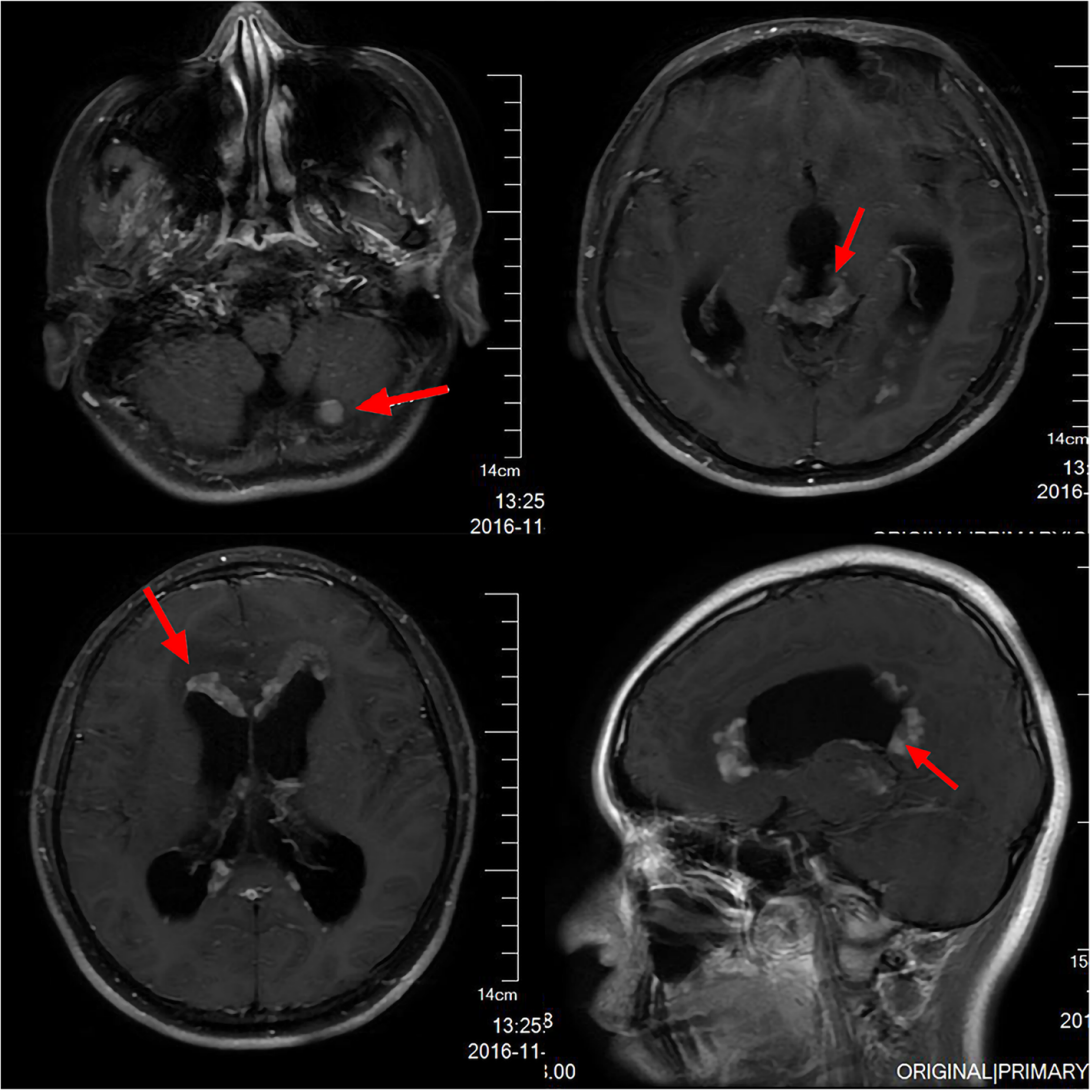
An MRI examination revealed hydrocephalus due to multiple relative space-occupying lesions in the pineal region, suprasellar region, lateral ventricles, and cerebellum (red arrow marks the lesions)
